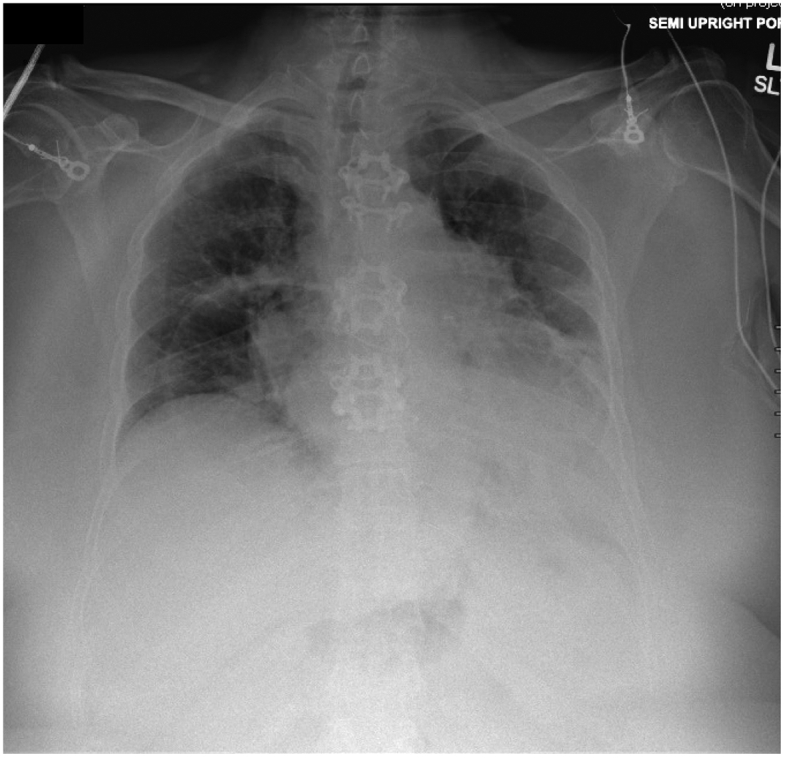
Chest X-ray obtained after surgery showing full resolution of the anterior diaphragmatic defect.
